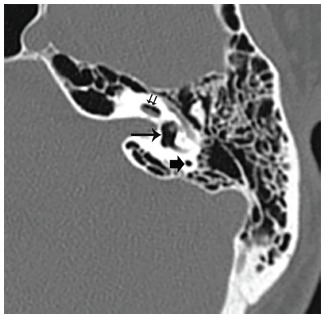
An axial cut of temporal bone computed tomography shows air in the left vestibule (long arrow), the posterior semicircular canal (short arrow), and the scala vestibuli compartment of the cochlear basal turn (short double arrows). Air was also seen in the lateral and superior semicircular canals (not shown).
